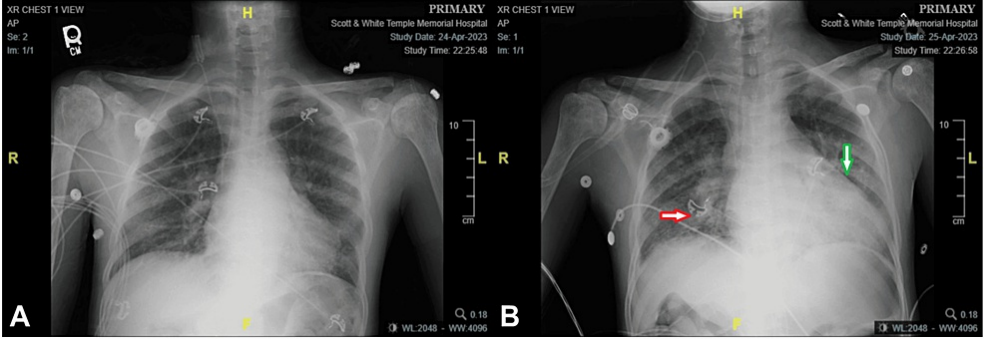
Chest X-ray demonstrates the development of interstitial and alveolar opacities in both mid to lower lung zones (red arrow) and enlarged cardiac silhouette (green arrow) in day 2 (B) in comparison to day 1 (A)
